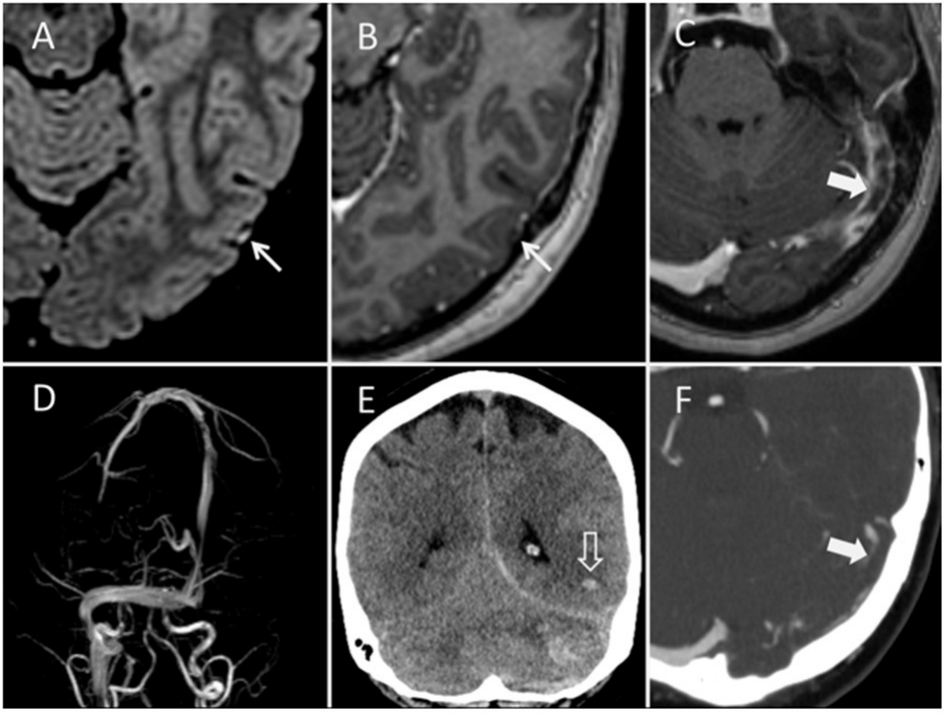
MRI, CT, and CT venography of patient 3. Thrombosis in a cortical vein (thin arrows) is possible to spot with high signal intensity on axial FLAIR (A) and filling defect on contrast enhanced axial T1-MPRAGE (B). Corresponding temporo-occipital hemorrhage (open arrow), cerebellar hemorrhage and high attenuation from thrombus in transverse and sigmoid sinuses can be seen on coronal CT (E). 3D phase-contrast MR venography demonstrates loss of flow signal in left transverse and sigmoid sinus (D). The thrombus in sigmoid sinus (thick arrows) is also evident on contrast enhanced axial T1-MPRAGE (C) and CT venography (F).
